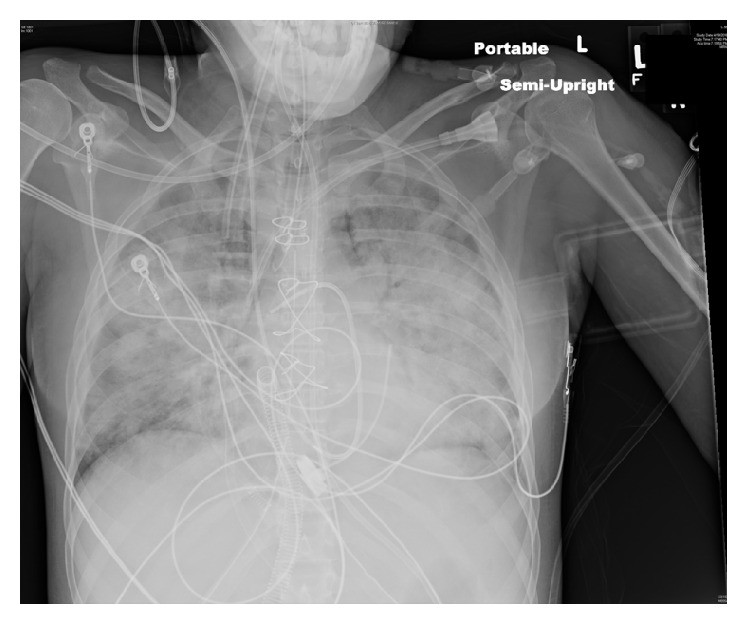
Repeat chest X-ray after intubation shows worsening bilateral aeration with developing patchy infiltrates.
